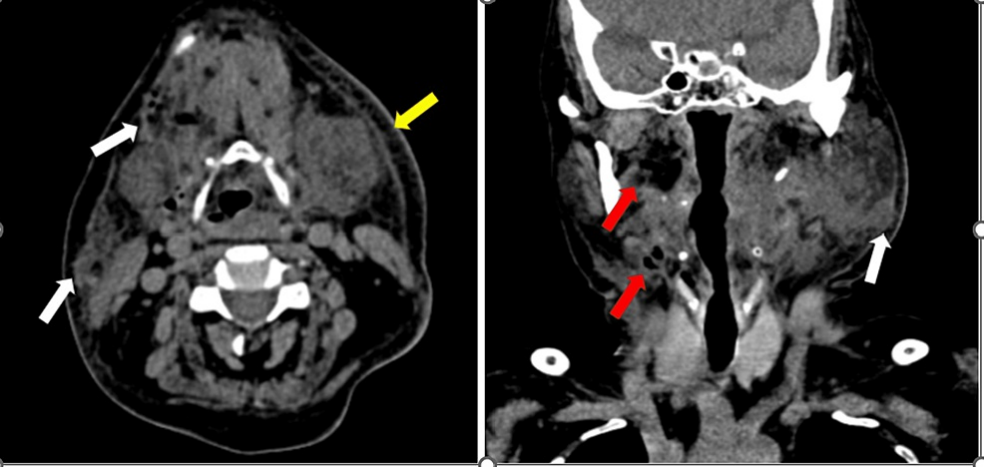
(Case 4) Plain CT neck (axial and coronal section) reveals an ill-defined hypodense fluid collection with multiple air foci involving the bilateral submandibular space platysma (white arrows), floor of mouth, and right parapharyngeal fat space (red arrows). Subcutaneous edema and stranding is also noted (yellow arrow).
